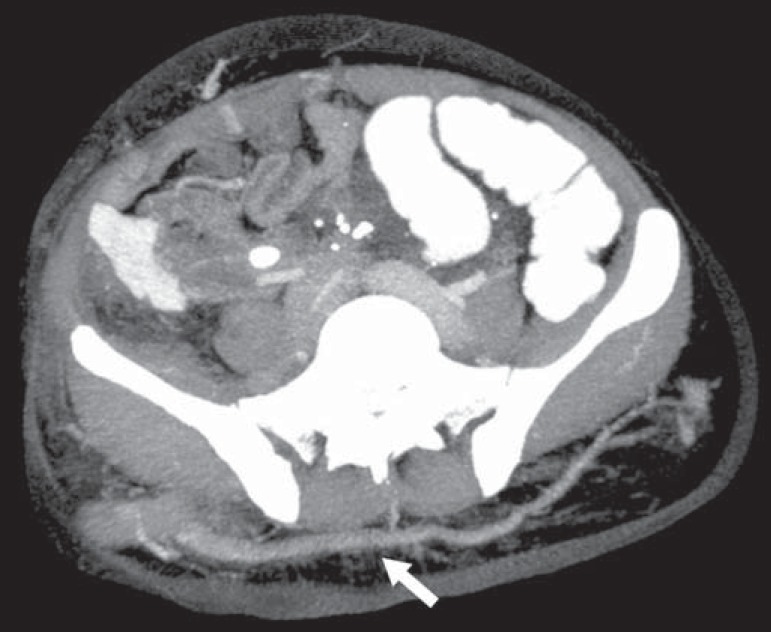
Klippel-Trenaunay syndrome. Axial maximum intensity projection CT
